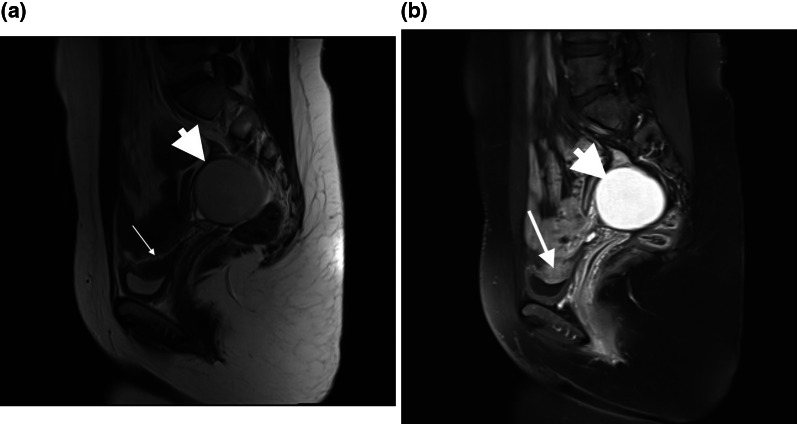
a T2 weighted sagittal MRI image without contrast showed a hyposignal plaque on the dome of the bladder and a typical endometrioma in the ovary (hyposignal in T2). b T1 weighted sagittal fat-suppressed image with contrast in the same patient showed enhancement of the plaque proved to be endometriosis in surgery, endometrioma typically hypersignal in T1
